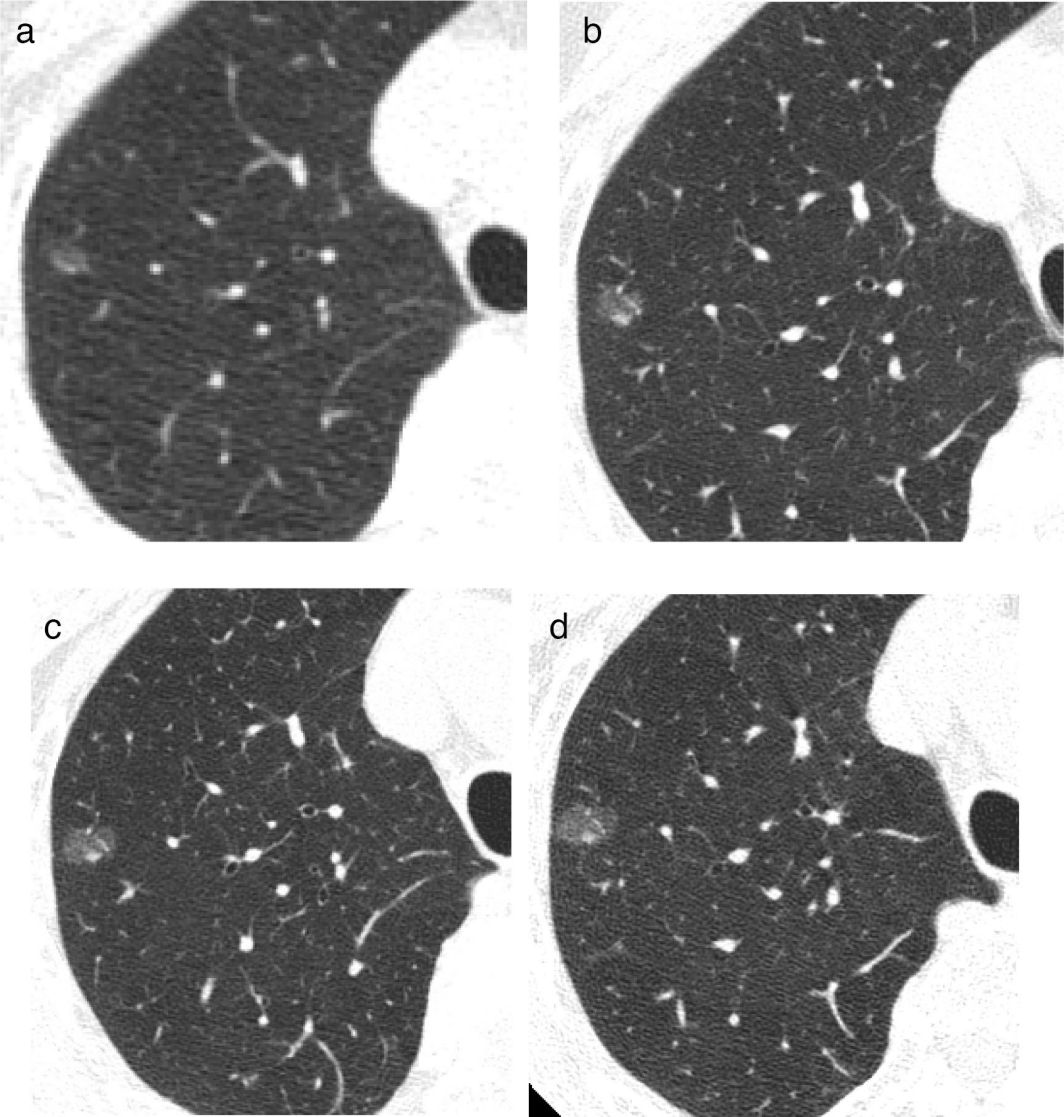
Minimally invasive adenocarcinoma. Low-dose CT screening image (a) Shooting conditions: CTDIvol = 0.6 mGy. Slice thickness: 2.5 mm; sequential approximation reconstruction (model-based iterative reconstruction: MBIR) (WW WL) = (1,200–, 600). The low-dose CT screening image (a) reveals a faint solitary nodule measuring 7 mm in the right upper lobe. b–d Thin-section CT (axial image) 1 month after screening (b), 2 years later (c), and 4 years later (d); slice thickness: 0.625 mm (WW WL) = (1,200–, 600). One month after screening, the thin-section CT (b) reveals a lesion with ground-glass predominance in the right upper lobe, measuring 8 mm in total diameter with a solid component diameter of 3 mm. Two years later (c), the lesion measures 9.5 mm in total diameter, with the solid component diameter remaining at 3 mm. Four years later (d), the lesion measures 13 mm in total diameter, with the solid component diameter increasing to 4 mm. Owing to the total diameter increasing by more than 2 mm, surgical resection was performed for definitive diagnosis. Pathological examination revealed fibrotic foci measuring 4 mm, resulting in a diagnosis of minimally invasive adenocarcinoma (pT1miN0M0)
